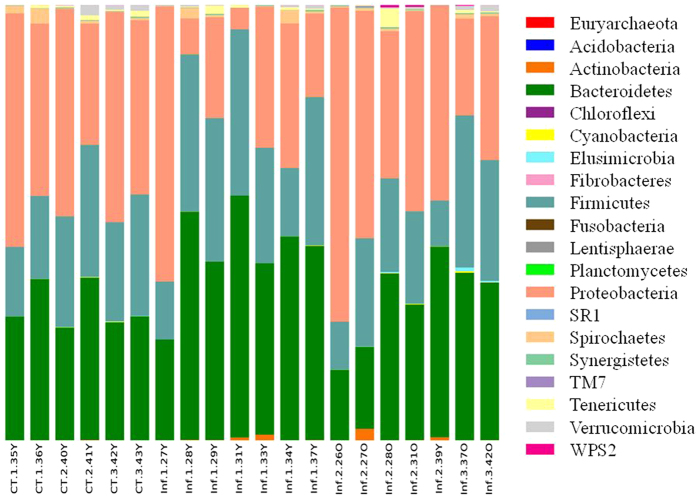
Phylum-level microbial composition in the caprine abomasal microbiome. CT: Uninfected Controls; Inf: Infected.
